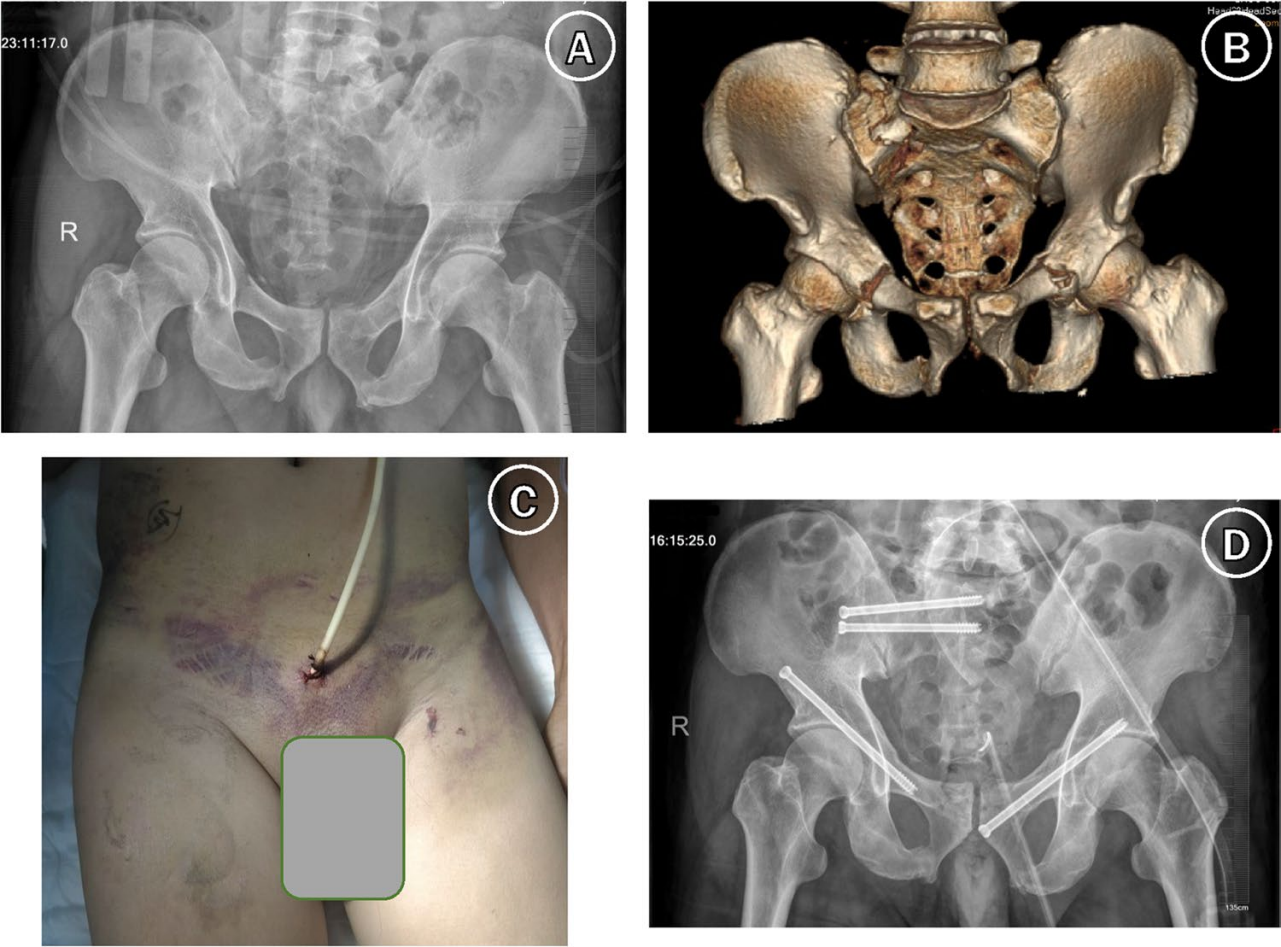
A 47-year-old man had an open nondisplaced pelvic fracture treated with RA. (A) Preoperative anteroposterior pelvic X-ray image. (B) Preoperative three-dimensional CT image. (C) The case was complicated with urethral injury, lung contusion, and fractures of the left tibia and fibula and right ankle. Emergency cystostomy was performed. (D) The patient was treated with percutaneous S1- and S2-level iliosacral screw and anterograde/retrograde pubic ramus screws fixation with the TianJi Robot system. He could sit up or turn over in bed after the operation, and urethral repair was successfully performed later
